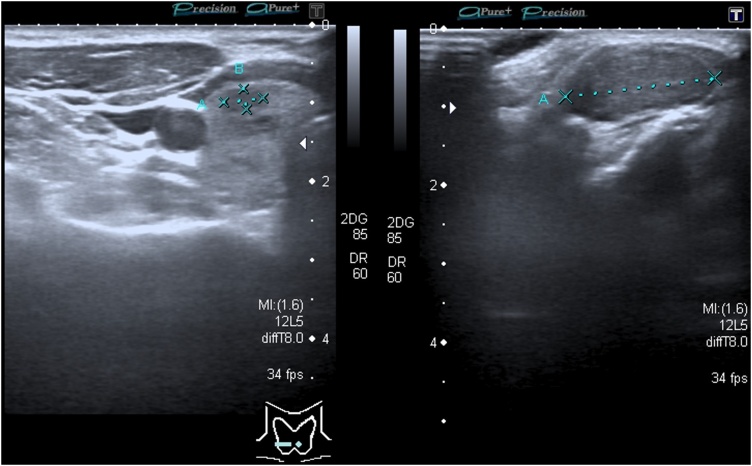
Ultrasonography of the neck solid hypoechogenic, slightly vascularised tissue 19 × 17 × 9 mm.
